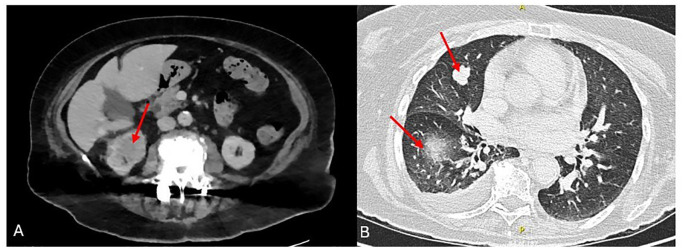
Computed tomography showing primary renal mass (A) and metastatic lung nodules (B) at diagnosis.
